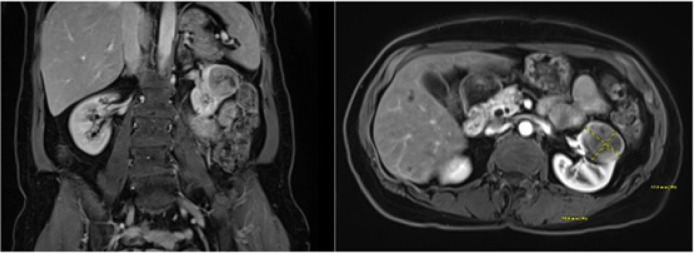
MRI of the abdomen with T1-weighted images in coronal and axial views.
